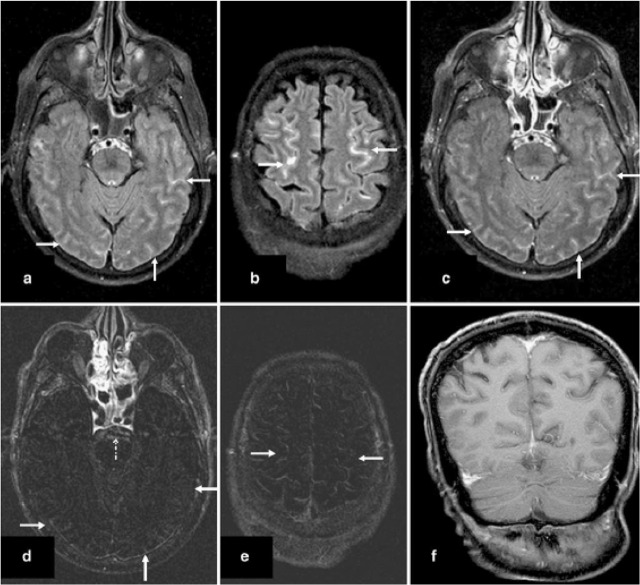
Sulcal hyperintensity in a patient with meningitis was not enhanced after subtraction of fat-suppressed SPIR/FLAIR images. Diffuse hyperintensity (arrows) in the cerebral sulci on FLAIR images before (a, b) and after (c) contrast (3-tesla). Subtraction images do not show the meningeal enhancement (d, e; arrows). On T1-W image with contrast, no meningeal enhancement is seen (f). After subtraction, the artifact of CSF flow in the prepontine cistern has been omitted (d, dashed arrow) (McKinney et al., 2006).
